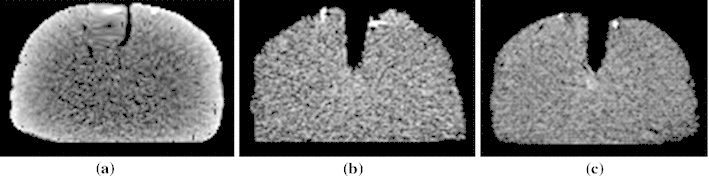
Warped images updated by a the traditional back-interpolation algorithm and b the modified back-interpolation algorithm. c Intraoperative images acquired by CT
